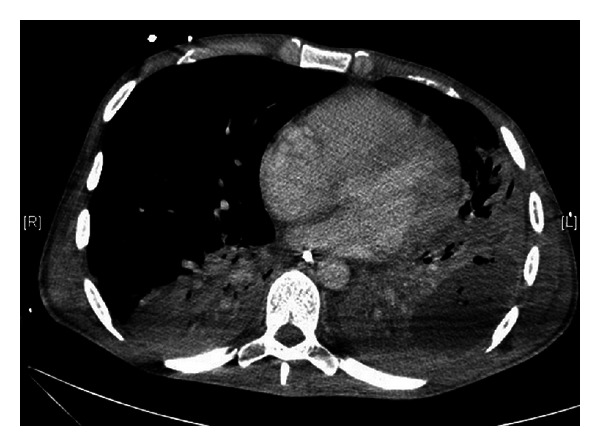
Thoracic CT scan revealing a voluminous lung abscess on the left pulmonary upper lobe on hospital day 35.
